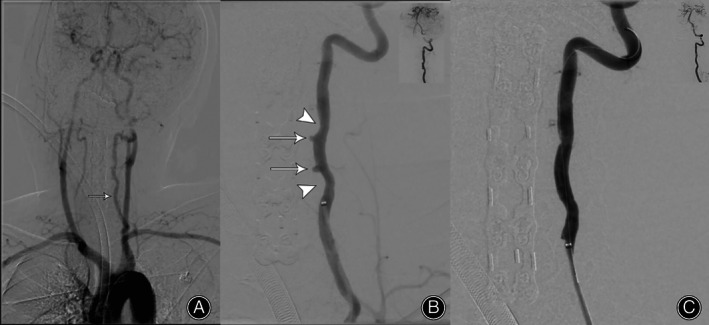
Intraoperative angiography showing that the left vertebral artery was dominant (A, arrow); two vertebral artery pseudoaneurysms (B, arrow) are evident in the left ruptured transverse foramina at C3 and C4 with no contrast extravasation. There are two incurved notches (B, arrowhead) next to the two pseudoaneurysms, indicating that the ruptured medial transverse foramina wall is filled with bone wax. The intraoperative fluoroscopy radiograph shows that after the stent was fully deployed, the two pseudoaneurysms were completely resolved, with no significant stenosis and good flow in the left VA (C)
